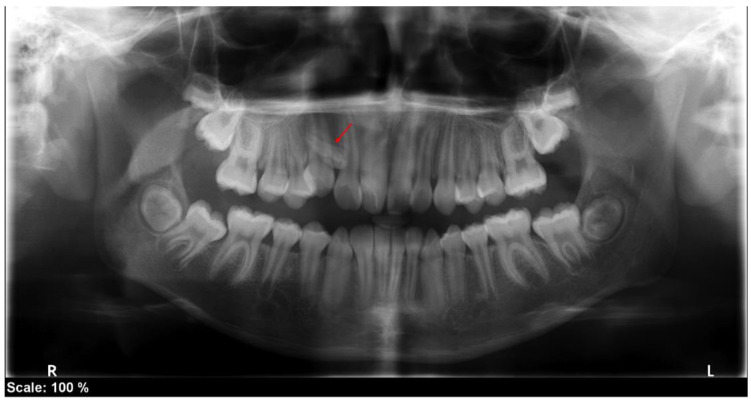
Panoramic X-ray. 2021, five years after the first surgery. Presence of a radiopaque element with a denticle shape in relation to teeth 12 and 13. Red arrow = odontoma.
